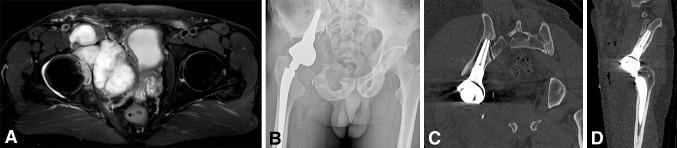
A–D (A) Case discussion of a 44-year-old male patient. T2-weighted MR image in the transverse plane shows a bulky mass, originating in the right acetabulum and infiltrating the hip joint. CT-guided biopsy showed a Grade 2 chondrosarcoma. (B) Conventional radiograph displaying the situation after Type 2–3 internal hemipelvectomy and subsequent reconstruction. Reconstruction was performed with an uncemented LUMiC^®^ stem (75 mm long, 10-mm core diameter), a 54-mm outer diameter HA-coated cup, and an uncemented Mallory-Head total hip prosthesis (Biomet, Warsaw, IN, USA) with a 28-mm femoral head. (C) CT scan displaying the position of the LUMiC^®^ stem in the coronal plane with its tip close to the sacroiliac joint. (D) CT scan displaying the position of the LUMiC^®^ stem in the sagittal plane.
